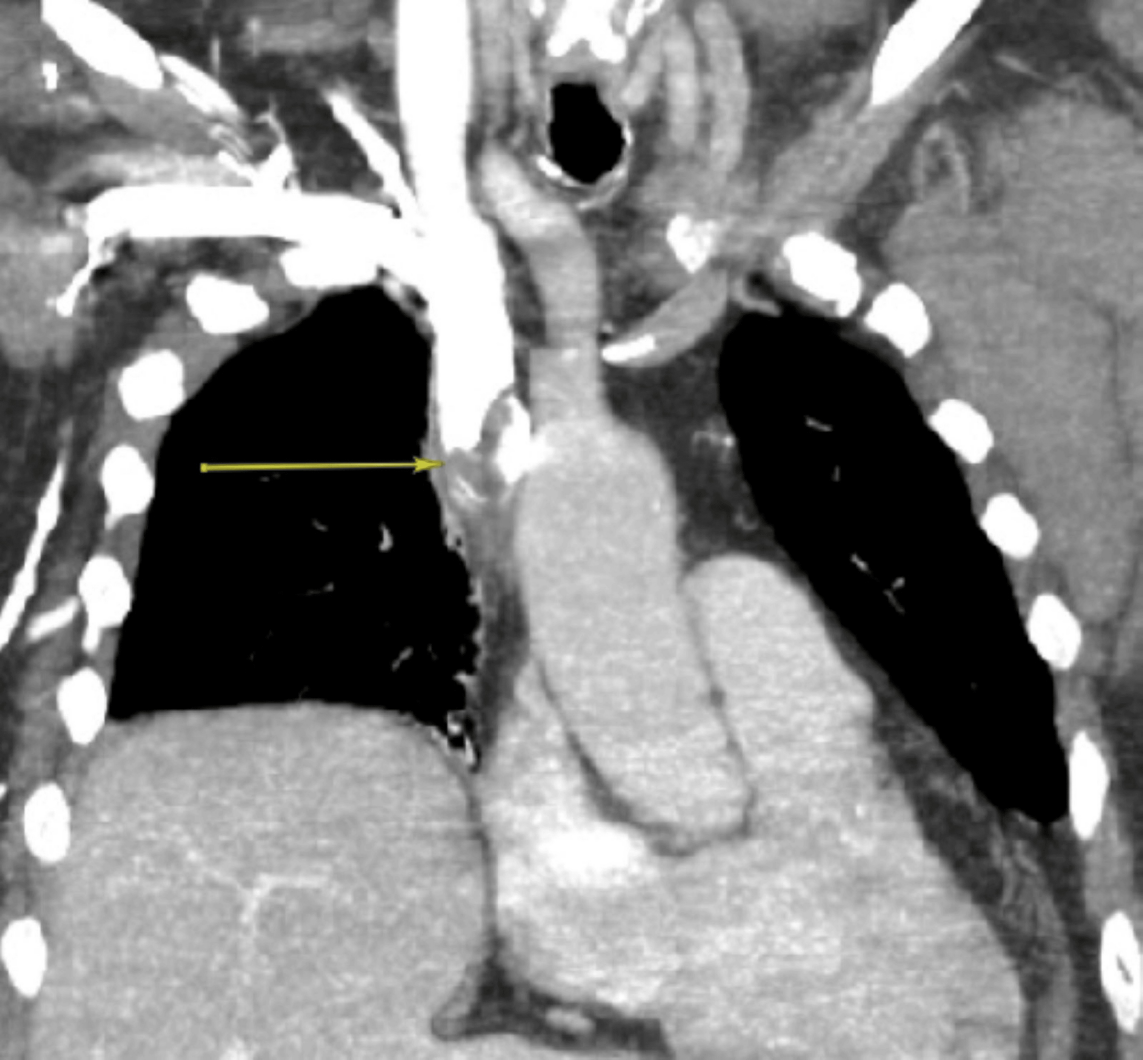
CTA thorax with IV contrast (coronal view) demonstrating catheter-associated thrombosis in the SVC (yellow arrow) suspected with irregular filling defect extending to the superior cavoatrial junction with associated prominent collateral vessels in the right upper mediastinum and supraclavicular fossa. CTA, computed tomography angiography; IV, intravenous; SVC, superior vena cava.
